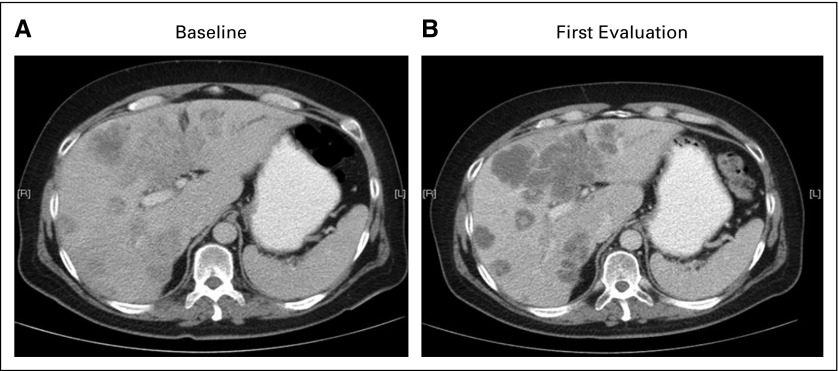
Stable disease in a patient with metastatic colorectal cancer during treatment. Computed tomography scans (A) before treatment and (B) at first evaluation (8 weeks). The on-treatment scan indicates tumor necrosis characterized by homogeneous hypo-attenuation and sharp tumor-liver interface (Data Supplement).
